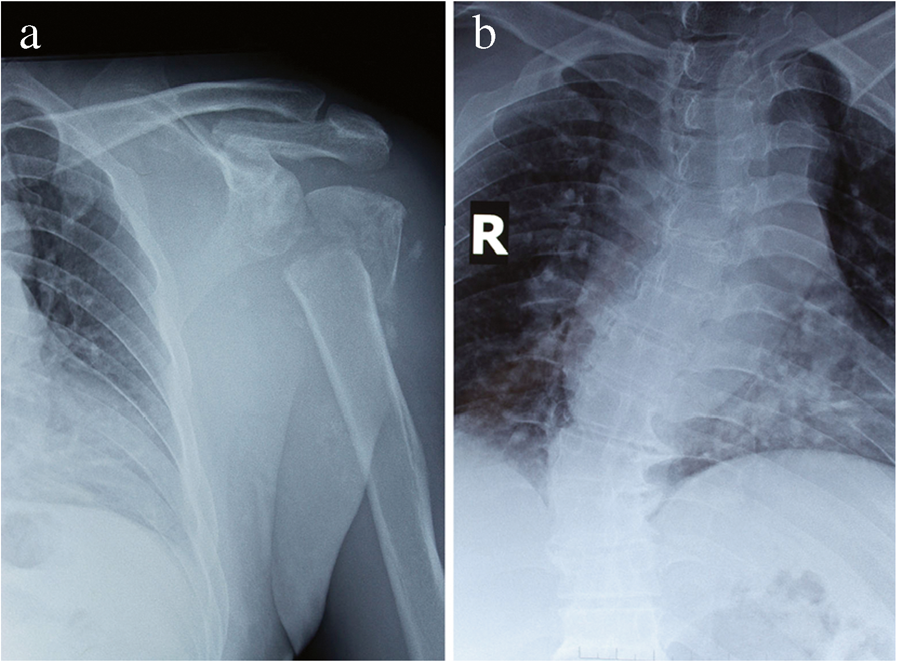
X-ray of this case. The surface of articulation of humerus was conspicuous rough, the head of humerus was disappeared completely (a), vertebrate column showed that the right lateral curvature of the lower thoracic vertebra (b).
